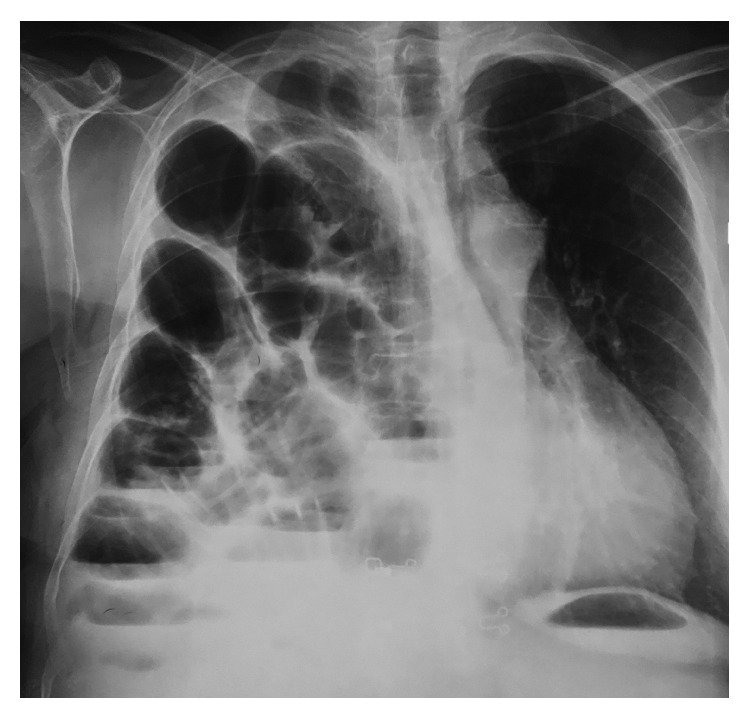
Chest X-ray performed immediately after admission to the Emergency Department. A large part of the small intestine has been transported to the right hemithorax causing functional right pneumonectomy. This image is in accordance with the clinical findings of the patient, which suggested acute respiratory distress syndrome.
